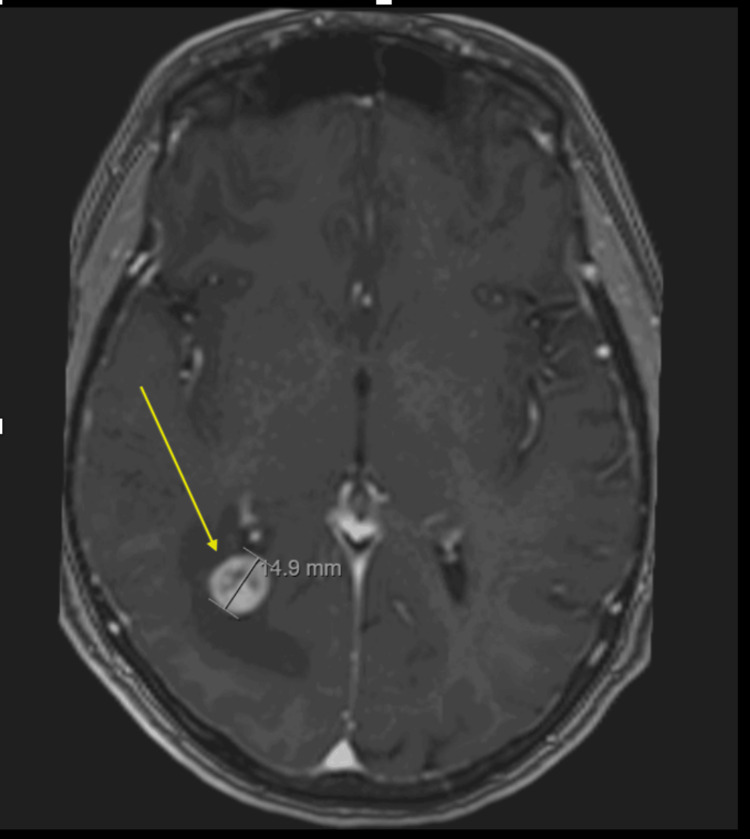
MRI head with contrast demonstrating a presumed cerebral metastasis with surrounding oedema.
